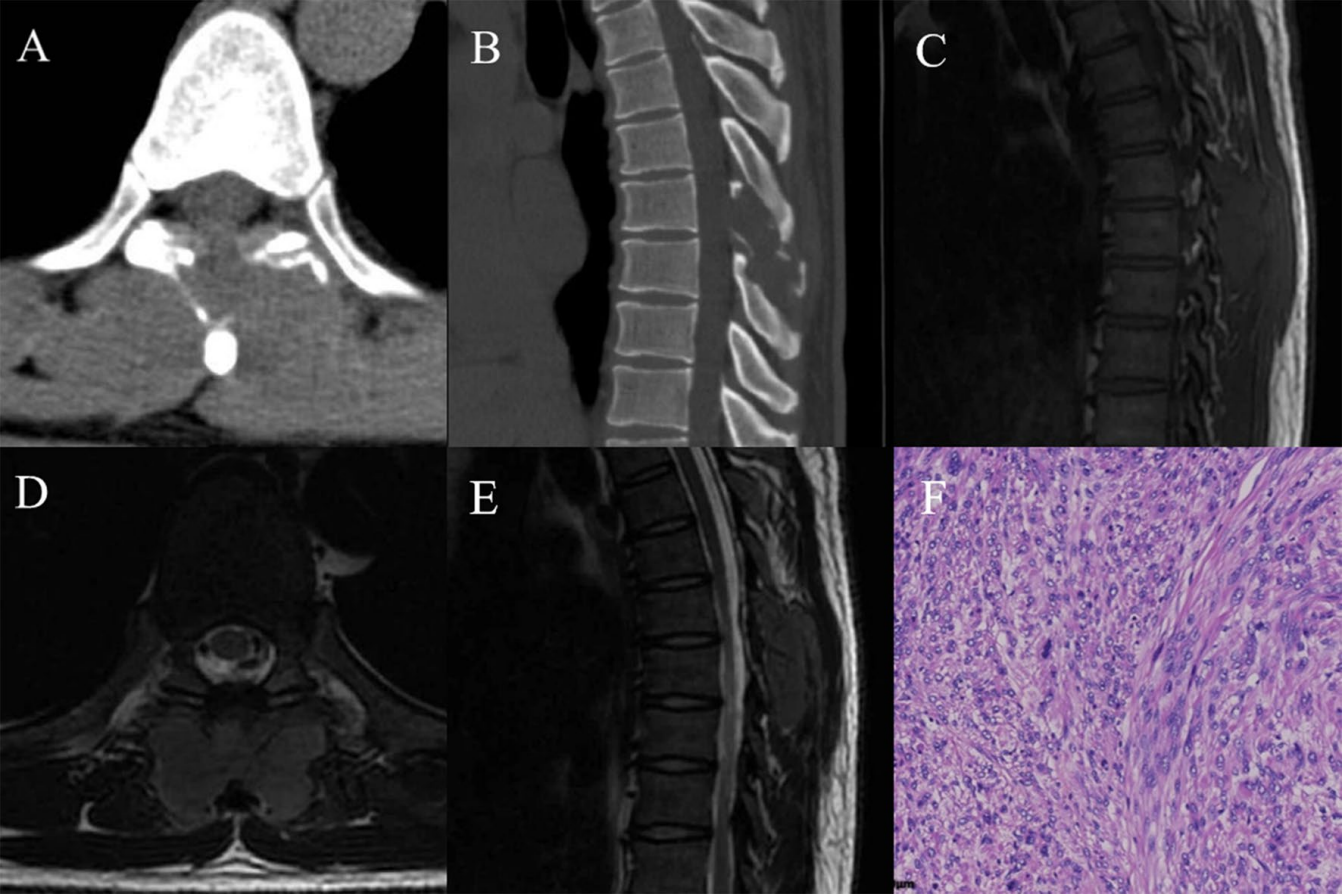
A 30-year-old male with T7–T8 primary leiomyosarcoma. A Axial computed tomography (CT) showed an ill-defined isodense mass in the posterior elements of T7–T8; B Sagittal CT bone window showed bone destruction in the posterior elements of T7–T8; C T1 weighted imaging (T1WI) in sagittal view showed an isointense mass; D T2 weighted imaging (T2WI) in axial view showed a lobulated mass; E T2WI in sagittal view showed an isointense mass; F the diffuse spindle cell hyperplasia confirmed to be a feature of interstitial tumors. The tumor cells were visibly atypical, and the nuclear fission rate was high (HE, ×400)
